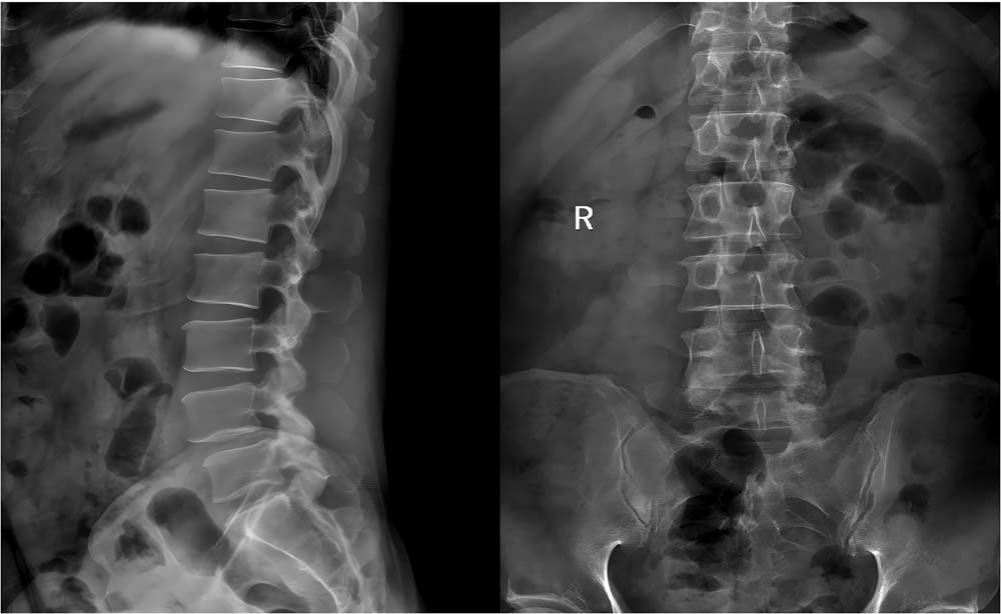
Preoperative anteroposterior and lateral radiographs of the lumbar spine.
